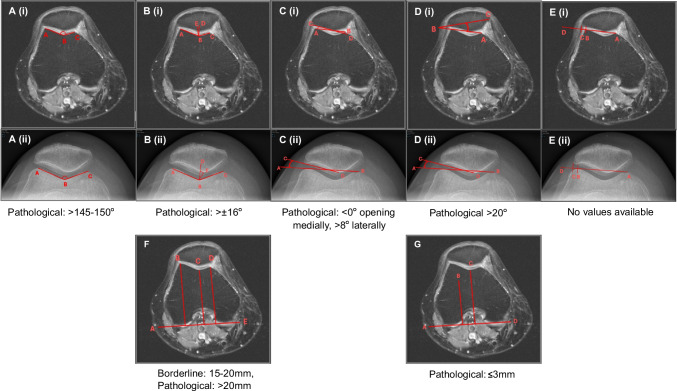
Radiological measurement methodology for patellofemoral joint measurements using axial MRI and skyline X-ray images. A Sulcus angle (SA): (i) MRI and (ii) X-ray: SA is angle < ABC, formed by Lines AB (lateral femoral condyle summit to the deepest trochlear groove) and BC (medial femoral condyle summit to the same point) (Brattstrom et al. [17]). B Congruence angle (CA): (i) MRI and (ii) X-ray: CA is angle < DBE, where Line BD bisects SA (< ABC), and Line BE extends to the lowest point of the patellar articular ridge. Medial orientation is negative, lateral is positive (Merchant et al. [18]). C Lateral patellofemoral angle (LPFA): (i) MRI and (ii) X-ray: LPFA is angle between Tangents AB (medial-lateral femoral condyle summits) and CD (lateral patellar facet) (Laurin et al. [16]). D Patella tilt angle (PTA): (i) MRI and (ii) X-ray: PTA is angle < ABC, formed by Tangents AB (femoral condyle summits) and BC (patella’s transverse axis). Lateral tilt is positive, medial tilt is negative (Grelsamer et al. [19]). E Lateral patella shift (LPS): (i) MRI and (ii) X-ray: LPS is calculated as (BC/AB) × 100%, where AB is femoral condylar width and BC is patellar displacement (Bito et al. [20]). F Trochlear depth (TD) (MRI only): TD is perpendicular distance from Line AE (posterior femoral condyles) to the lowest point of the trochlear groove, subtracted from the mean height of the medial and lateral trochlear facets (Pfirrmann et al. [21]). G Tibial tuberosity-trochlear groove (TT–TG) (MRI only): TT–TG is horizontal distance between the tibial tuberosity and trochlear groove, measured from perpendicular lines extending from the posterior femoral condyles (Line AD) to the tibial tuberosity (Line B) and the trochlear groove (Line C) (Dejour et al. [7])
